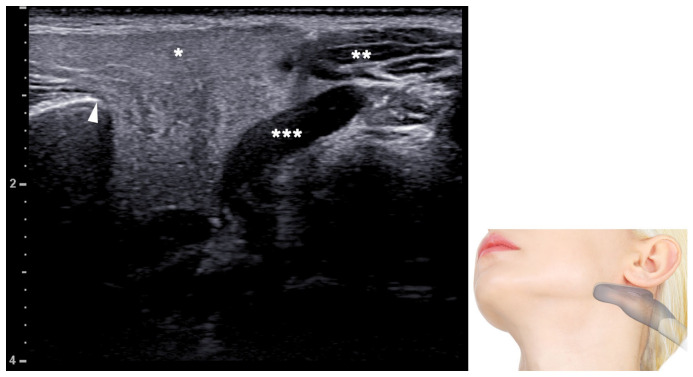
Ultrasonographic image of the region posterior to the angle of the mandible. An ultrasound scanner (HS70A; Samsung, Seoul, Republic of Korea) with a linear transducer (L3-12A) was used in this study. Arrowhead, angle of mandible; *, parotid gland; , sternocleidomastoid muscle; *, posterior belly of digastric muscle. Adapted from data provided by H.J. Kim, with permission [8].
